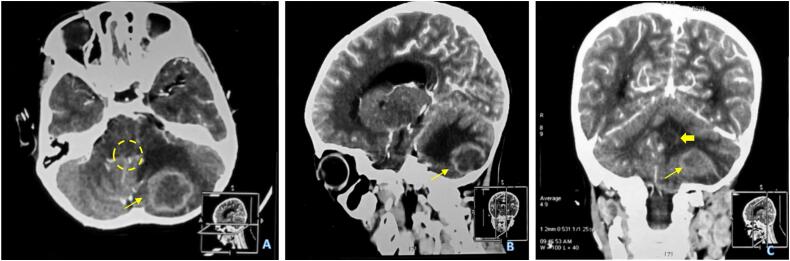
Head CT Scan with contrast revealing a multiple intra-axial mass of the posterior fossa. Axial cut of the head CT Scan (A), revealing a ring enhancing mass of the left cerebellum (thin arrow), causing mass effect and obliterating the fourth ventricle (dashed circle). Sagittal cut of the CT Scan (B). There's another intra-axial mass near the fourth ventricle causing distortion of the fourth ventricle (thick arrow). Non-contrast CT Scan revealed no contrast enhancement of the leptomeninges.
